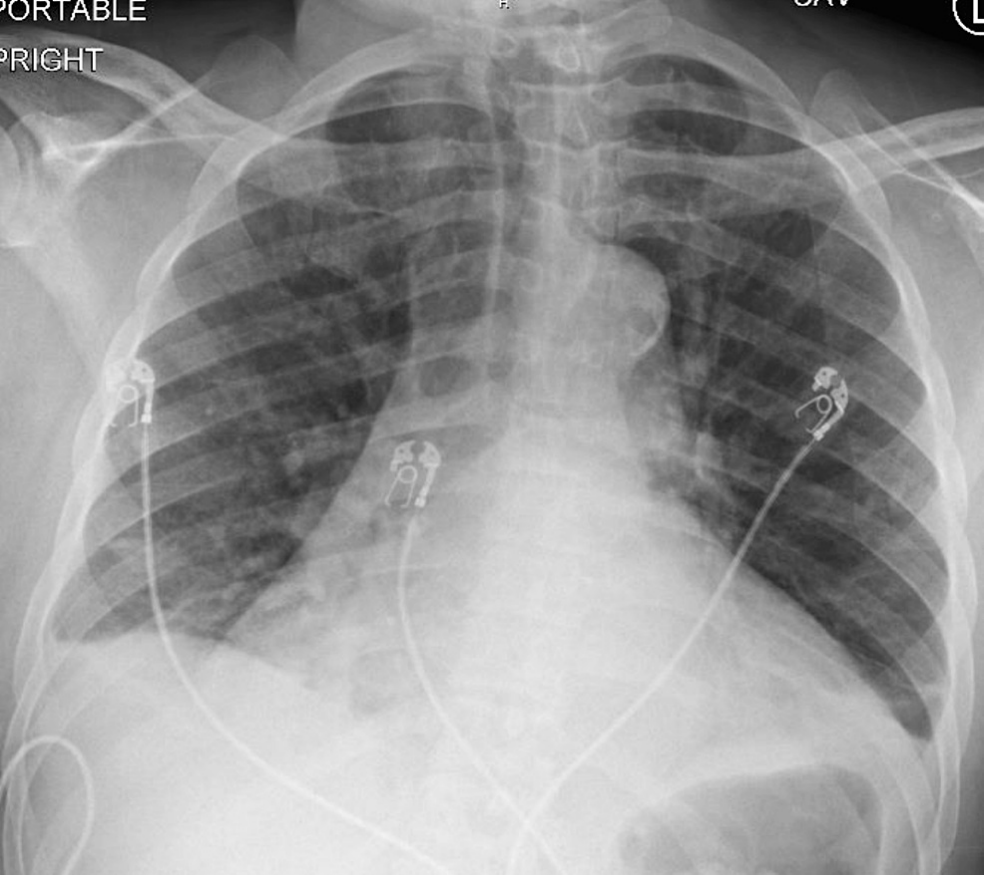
The chest radiograph shows small bilateral pleural effusions with mild bibasilar atelectasis.
